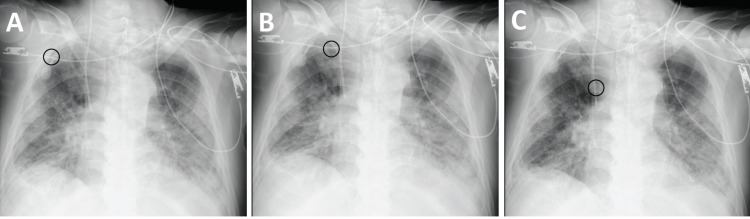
Chest radiograph showing the positions of the PICC tip (A) Placement of the tip in the subclavian vein. (B) Direction of the point of the tip toward the superior vena cava. (C) Placement of the tip in the lower half of the superior vena cava and the upper part of the right atrium. The images of (A) and (B) depict the application of torque while inserting the PICC. The black circle shows the positions of the PICC tip. PICC: peripherally inserted central catheter
